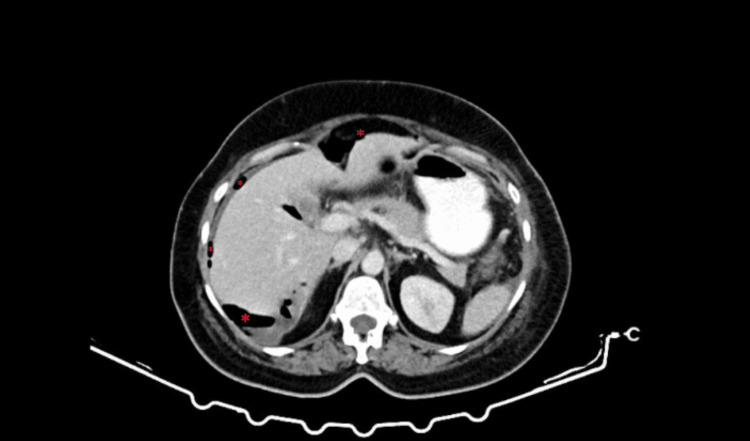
CT scan of the upper abdomen showing signs of fecal peritonitis and pneumoperitoneum caused by perforation of the small intestine. Red asterisks: air collections under the diaphragm (pneumoperitoneum).
